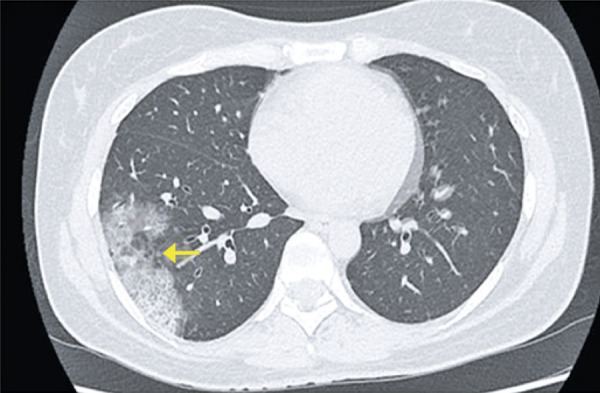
A 44-year-old patient with COVID-19, presenting headache, odinophagia, productive cough, dyspnea and myalgia for six days. Computed tomography showing ground-glass opacity in the periphery of the right lower lobe, associated with reticulations and interlobular septum thickening (crazy paving pattern)
